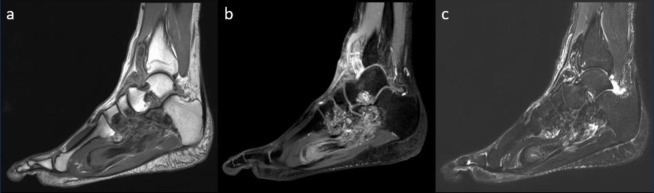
Left foot Magnetic Resonance Image (MRI) with contrast: shows an infiltrative soft tissue mass extending from distal pre-tibial level to the dorsal and plantar aspects of the midfoot showing extensive multifocal soft tissue and intra-articular involvement with tendinous, vascular encasement and causing multifocal bony erosions measuring roughly 15.5×7×10 cm (AP×TL×CC), a. It shows intermediate to hypointense signal on T1 weighted images, particularly peripheral low signal intensity, b. There is similar intermediate to hypointense signal on T2-weighted images with foci of hyperintense component, c. It shows heterogeneous post-contrast enhancement
